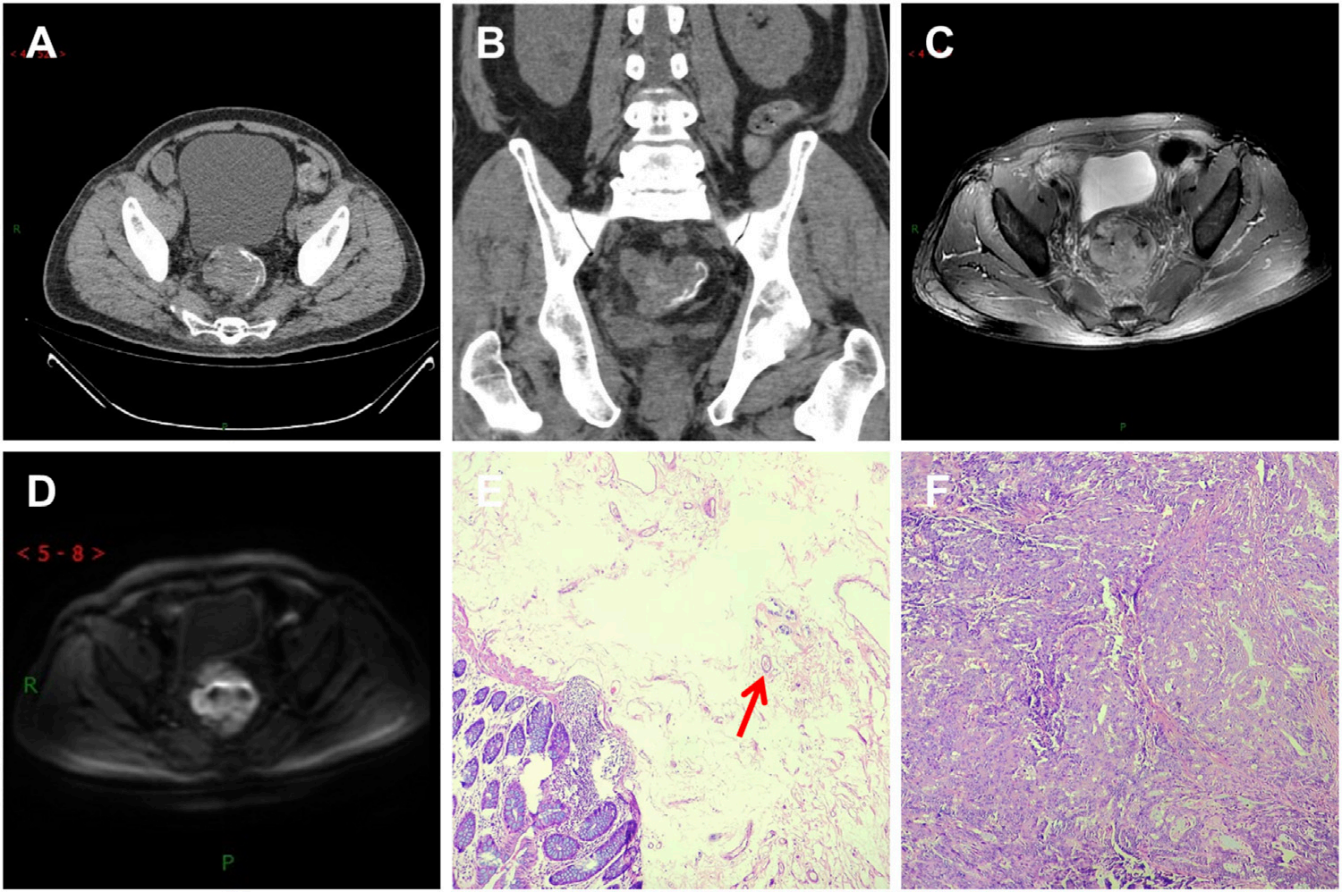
A patient with moderately differentiated adenocarcinoma of the rectum, with the tumor invading the entire intestinal wall and lymph node metastasis visible outside the intestinal wall (T3N1MX stage). (A) CT shows soft tissue mass shadows in the rectal area, linear calcification shadows in the submucosa of the intestinal wall, and lymph node shadows in the surrounding area; (B,C) T2WI transverse and sagittal images showed that the lesion involved all rectal walls and broke through the muscular layer. Burr-like and flocculent shadows were seen around the lesion. The boundary between the lesion and surrounding Mesentery fat was unclear. Slightly high signal lymph node shadows were seen in the rectal mesentery fat; (D) The DWI sequence showed significantly high signal intensity in the tumor; (E) In the pathological picture, the red arrow indicates the Schistosoma egg; (F) Tumor tissue.
